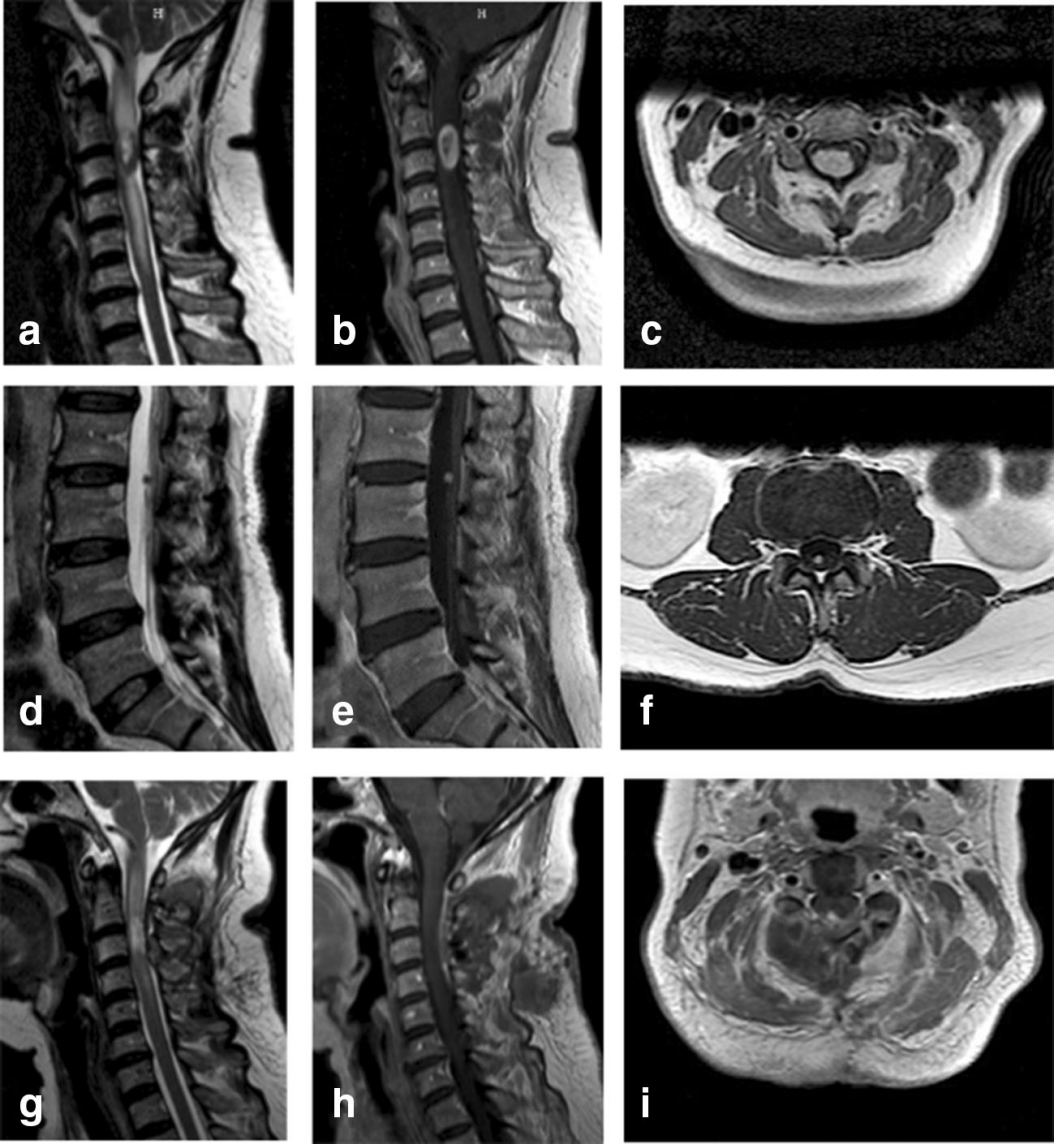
a–c Preoperative MRI of the cervical spine in sagittal (a, b) and transverse (c) slides. T_2_-weighted images show a hypo- and hyperintense intramedullary lesion at the level of C2/3 (a). T_1_-weighted images show a heterogeneous gadolinium-enhanced tumour in the sagittal (b) and transverse (c) slides. d–f Preoperative MRI of the lumbar spine in sagittal (d, e) and transverse (f) slides. T_2_-weighted images show a hypointense lesion at the level of L2/3 (d). T_1_-weighted images show a homogenous gadolinium-enhanced tumour in the sagittal (e) and transverse (f) slides. g–i Postoperative MRI of the cervical spine in sagittal (g, h) and transverse (i) slides confirming the complete tumour resection
